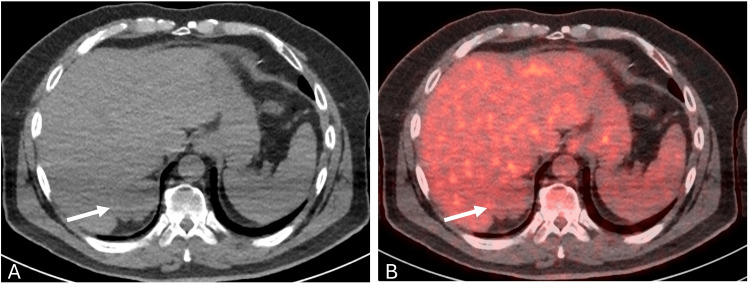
¹⁸F-FDG PET/CT images at the level of the right suprarenal lesion (A) Non-contrast CT image and (B) fused PET/CT image demonstrate a lobulated lesion noted posterosuperior to the right adrenal gland, closely abutting the adjacent segment 7 and right adrenal gland. The right adrenal gland shows low-grade FDG uptake (SUVmax of 3.1). ¹⁸F-FDG PET/CT, Fluorine 18-fluorodeoxyglucose positron emission tomography/computed tomography
